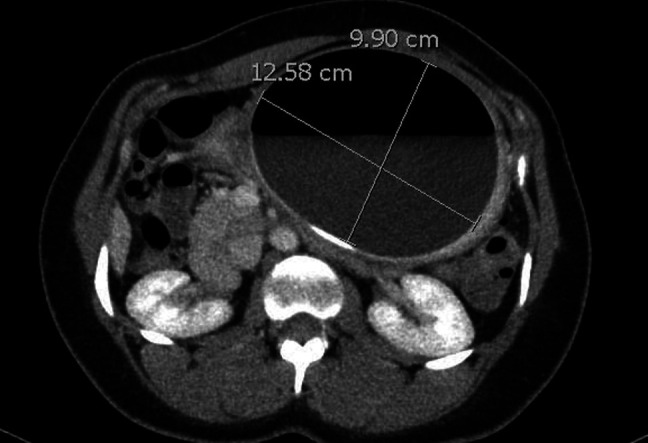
The hyperinflated intragastric balloon is vizualized within the stomach on computed tomography scan, measuring up to 12.58 cm in diameter.
